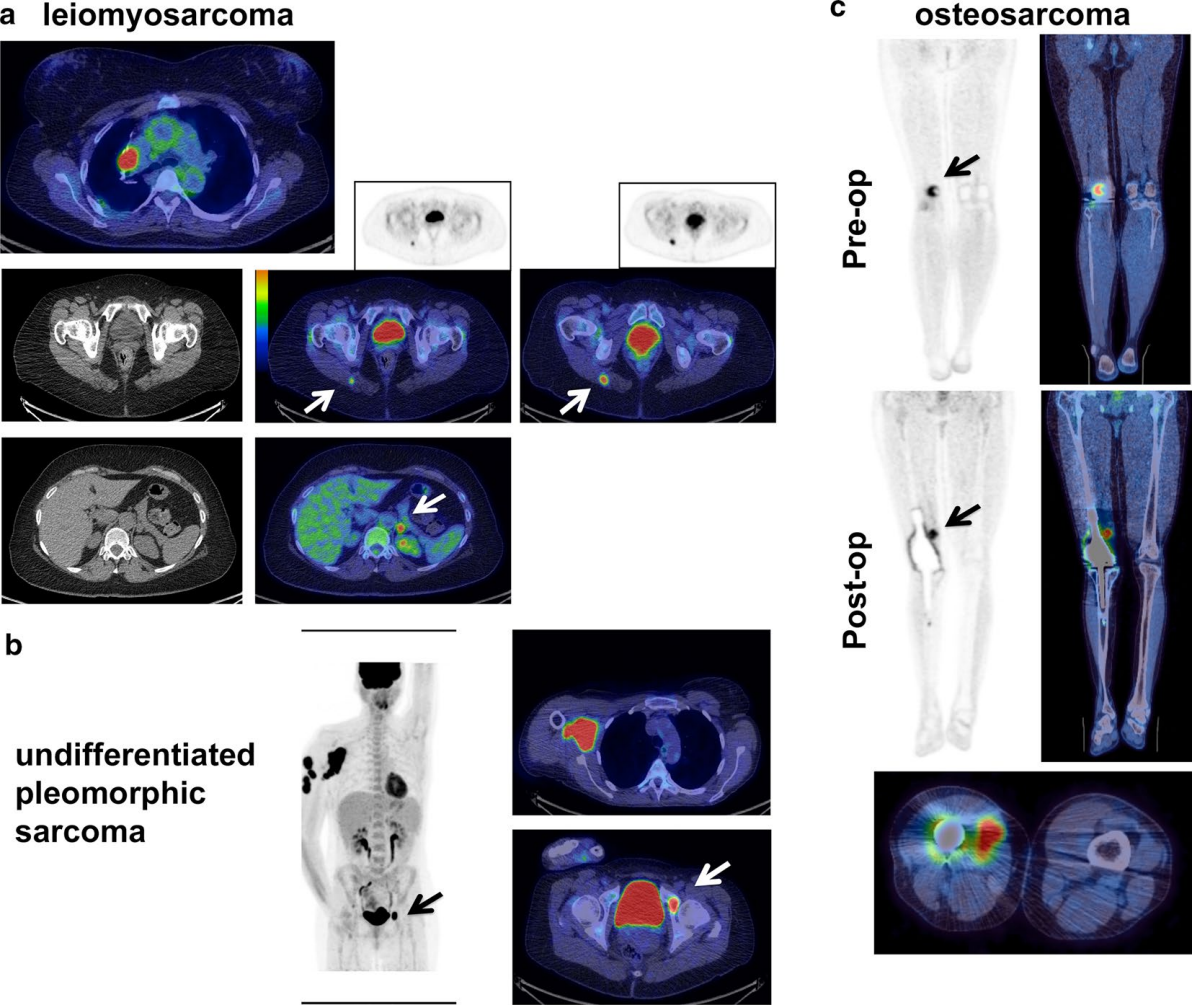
Examples of ^18^F-FDG PET and fused PET–CT images with added value detection of disease sites in sarcoma. All images are on an SUV scale of 0–6. a Case of a 57 year-old female with hilar lung metastatic leiomyosarcoma, but with occult metastatic sites (arrows; right buttock and left para-aortic region) not clearly evident on conventional CT scans. b Case of a 54 year-old female with undifferentiated pleomorphic sarcoma (UPS) with primary right axillary disease, but with an occult bone secondary in the pelvis (arrow) on PET–CT. c Case of a 23 year-old male with distal femur osteosarcoma post MAP chemotherapy (pre-op) and after reconstructive surgery and prosthetic replacement with a local recurrence (post-op). Arrow indicates FDG avid nodule of local recurrence close to the prosthetic margin not visible on CT
